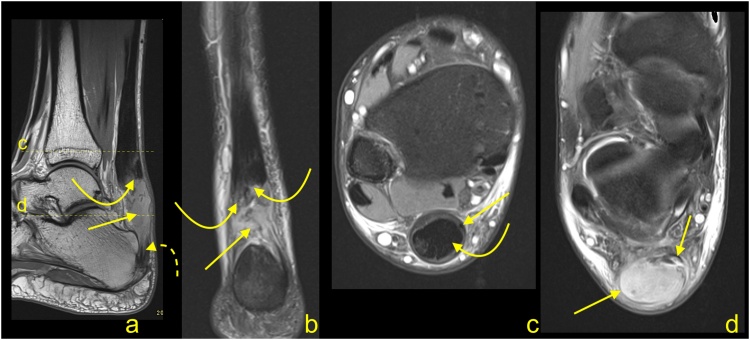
Total Achilles tendon rupture in the distal part 3 weeks ago. MRI showed that asymmetry of stumps is visible. The proximal stump is larger (curved arrow) while the distal stump is smaller (dashed curved arrow). The space between the stumps is filled with fluid (straight arrow). The plantaris tendon is preserved and contributes to the paratenon (dashed arrow). A- PD- weighted, b- T2- weighted with fat suppression, c and d- PD- weighted with fat suppression.
